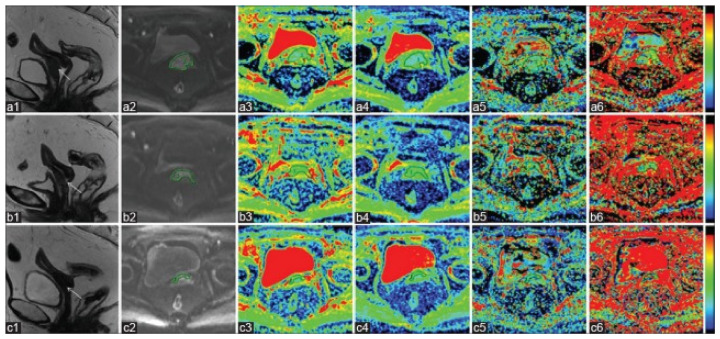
A 56-year-old woman with cervical squamous cell carcinoma (white arrow). Complete response after NACT. (a1–a6) Pre-NACT; (b1–b6) 3 weeks after the first NACT cycle; (c1–c6) 3 weeks after the second NACT cycle. (a1–c1) Sagittal T2-weighted images; (a2–c2) axial DWI with *b* = 800 s/mm^2^; (a3–c3) axial ADC maps; (a4–c4) D; (a5–c5) D*; (a6–c6) f values [28]. This figure was adapted from Wang et al. [28]. Originally published under the Creative Commons Attribution-Non-Commercial-ShareAlike 3.0 License. Permission for adaptation and reuse was also obtained from the original authors.
